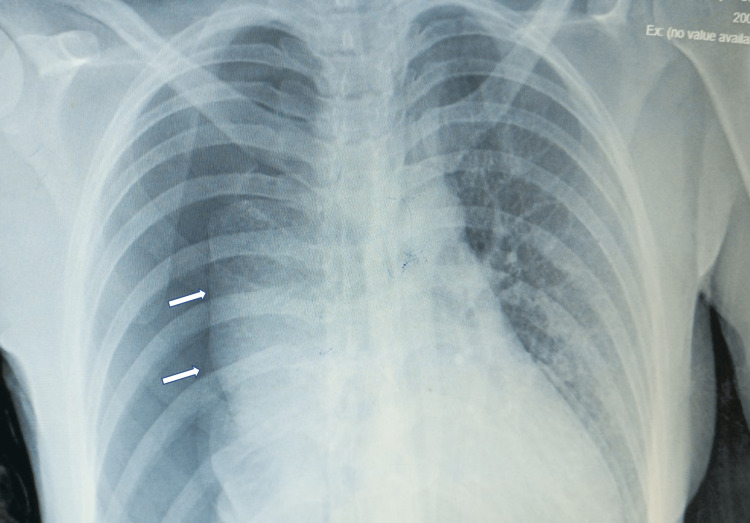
The chest X-ray shows the right pneumothorax. The two white arrows note the pneumothorax border.
